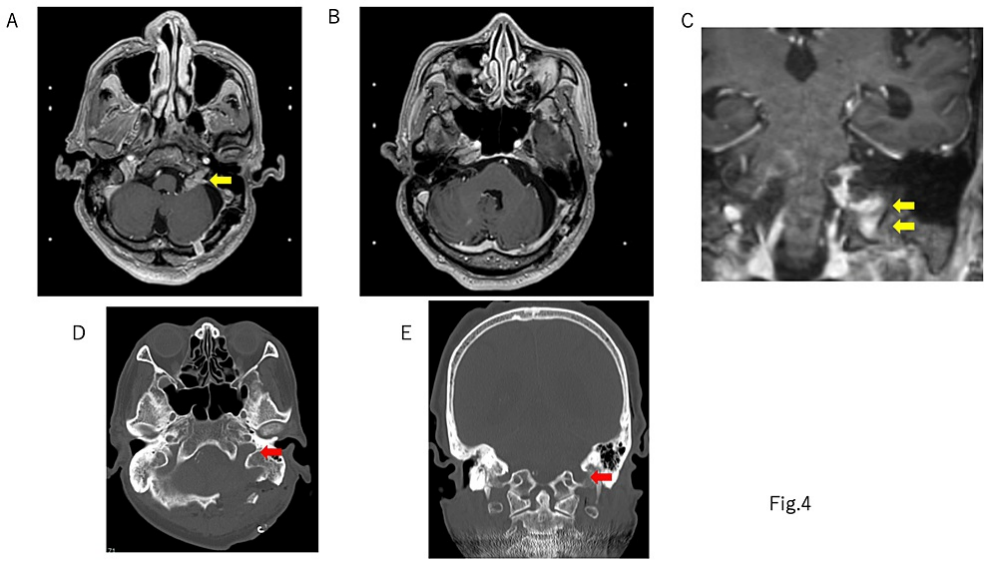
Postoperative gadolinium (Gd) MRI 80% of the cisternal portion of the tumor was removed, but the jugular foramen portion of the tumor was left. A, B: axial plane Gd MRI. C: coronal plane Gd MRI. D: axial plane CT bone image. E: coronal plane CT bone image. Yellow block arrow: residual tumor. Red block arrow: enlarged jugular foramen MRI: magnetic resonance imaging
